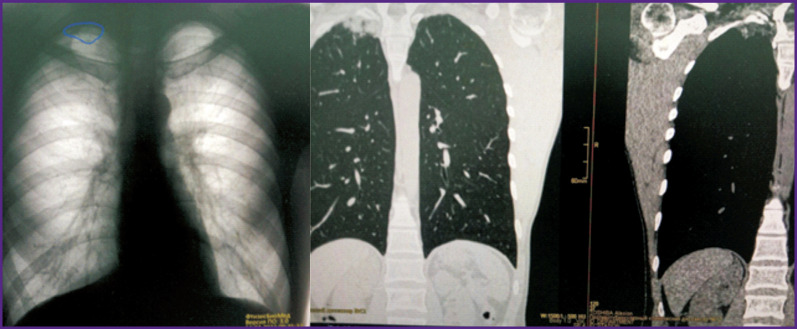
Detection of residual changes with the help of PhthisisBioMed artificial intelligence (*left*) and CT scan results (*right*)
